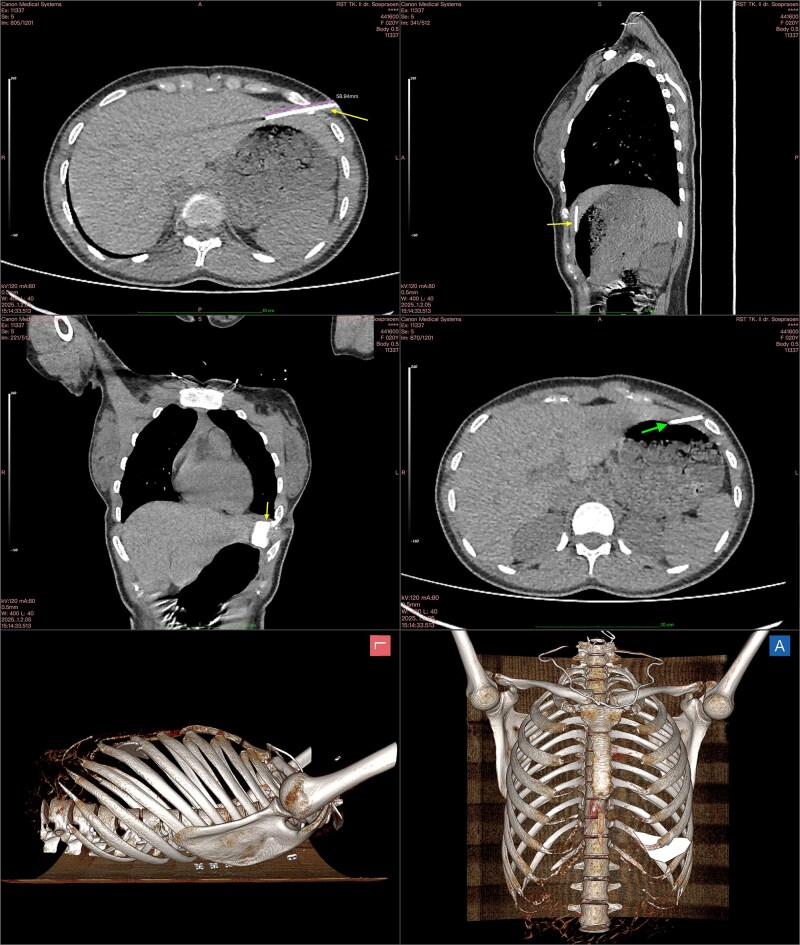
Chest CT with axial (top-left and middle-right), sagittal (top-right), coronal (middle-left) view, and 3D reconstruction with left (bottom-left) and anterior (bottom-right) view; FB is indicated by arrows and measured using a virtual ruler; an additional arrow identifies the suspected gastric perforation due to FB penetration (middle-right).
